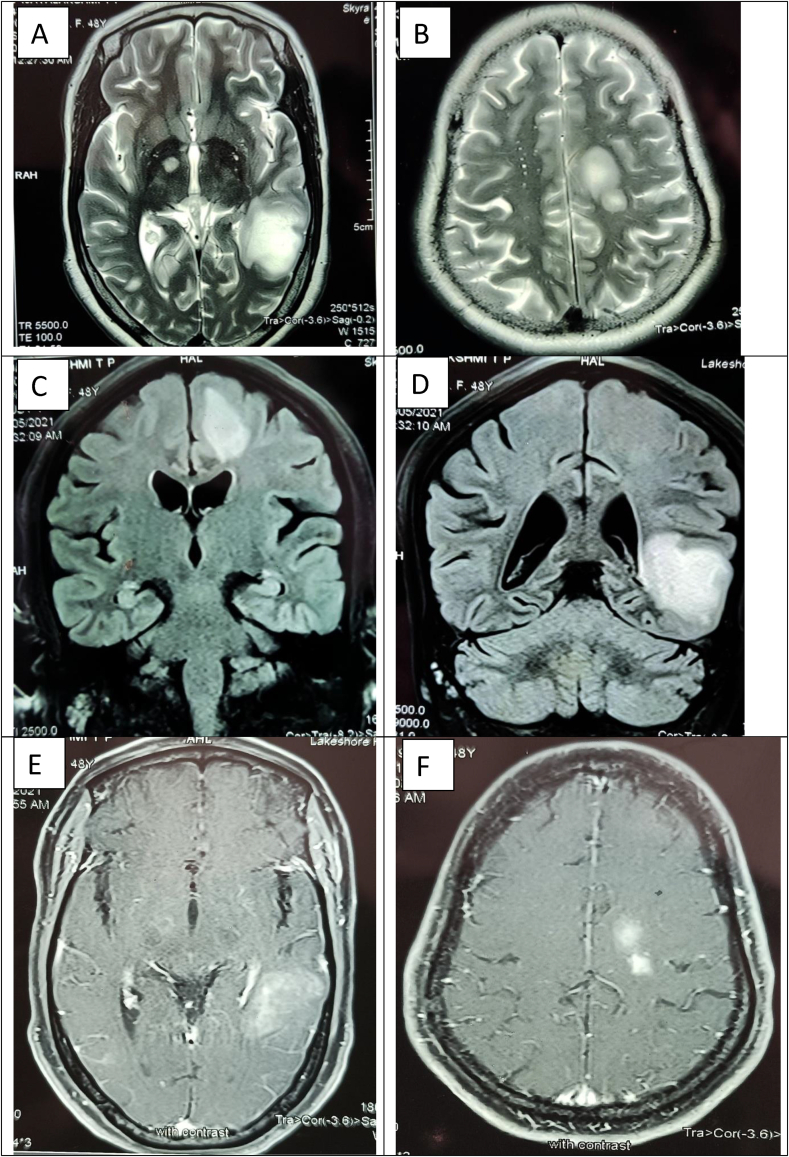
Brain magnetic resonance imaging (MRI) findings (Pre-treatment findings). Varying-sized peripheral rim-enhancing lesions with central T2 hyperintense signal & peripheral intermediate signal were noted in the bilateral posterior temporal, right centrum semiovale, left corona radiata, and the sub-lentiform internal capsule extending. The most significant lesion in the left posterior temporal lobe measures 3.4 x 3.0 cm. Minimal perilesional oedema was noted around this lesion with an open ring enhancement (open towards the cortex). The rest of the lesions show complete ring/heterogenous enhancement. Peripheral diffusion restriction was noted in the lesions with mild (A & B: axial view, C & D: coronal section, E & F – Post-contrast axial view).
